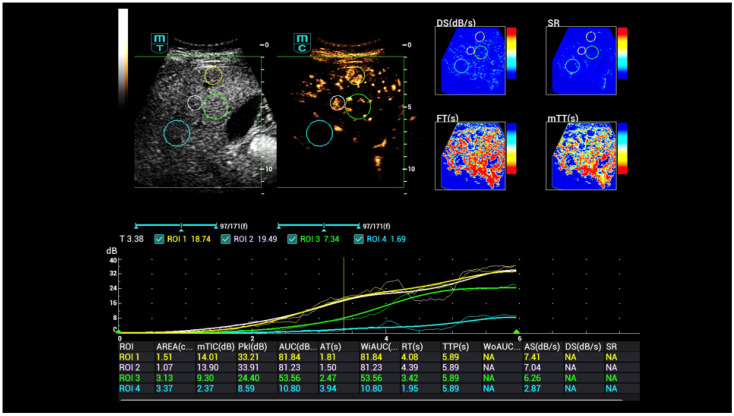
CEUS perfusion evaluation after injection of 1.4 mL ultrasound contrast agent with irregular hyperenhancement of the liver and early nodular enhancement of high-flow haemangiomas and micro-shunts. In the early arterial phase, there is strong contrast enhancement into the areas of micro-shunts.
